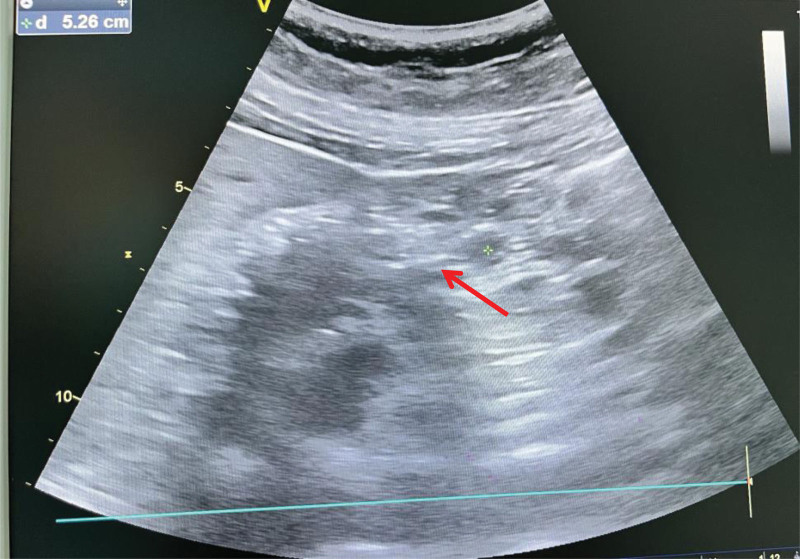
The “double track” sound image in the B-ultrasound pyloric imaging.
